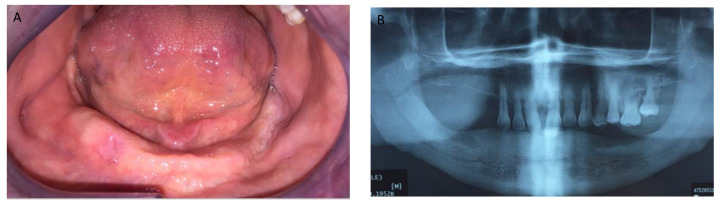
(A,B) Post-operative intra-oral and radiographic view at 6 month follow-up showing healed soft (A) and hard (B) tissues.
